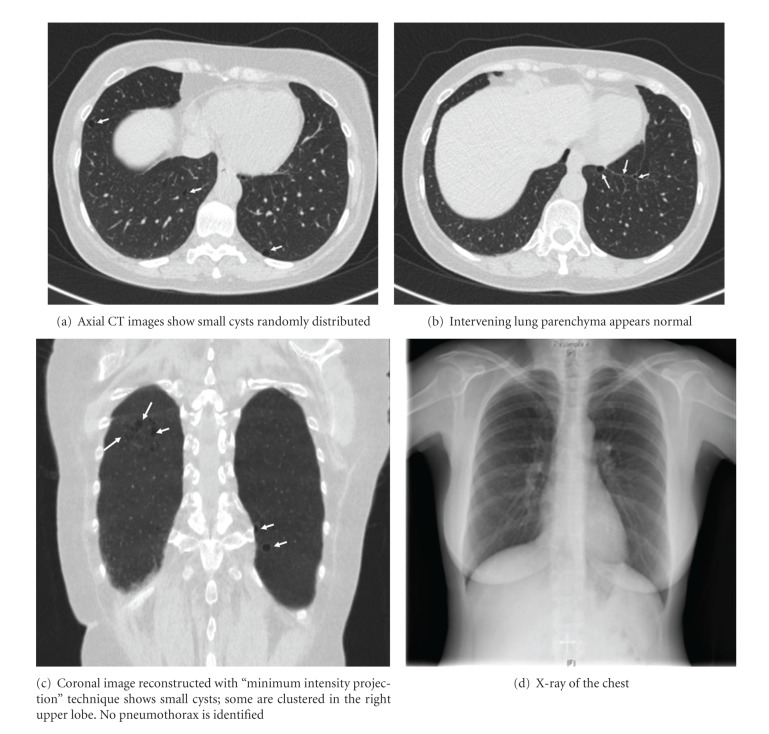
Preoperative CT scan of the thorax (a–c) and chest X-ray (d).
